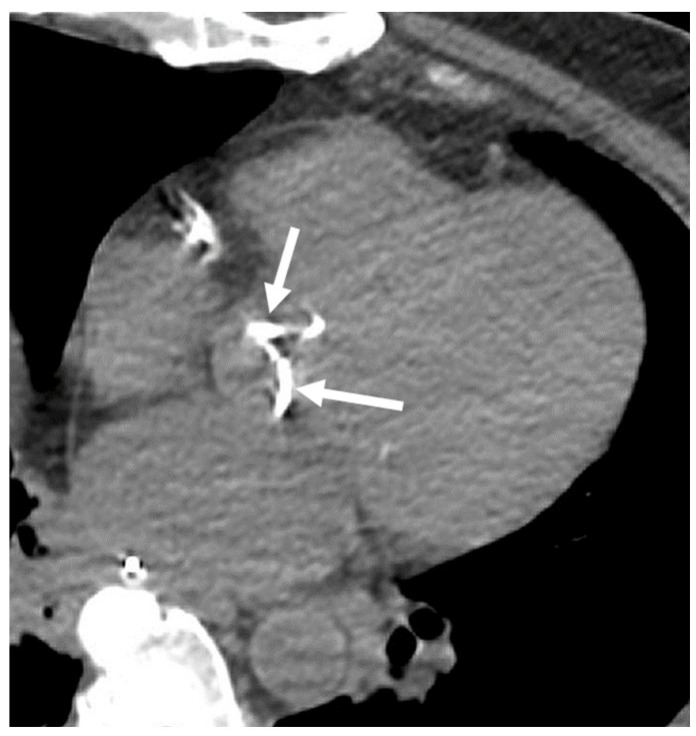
The incidental finding of aortic valve calcification on a computed tomograph (CT). An axial CT of a 65-year-old male demonstrates calcification of the aortic valve leaflets (arrows).
